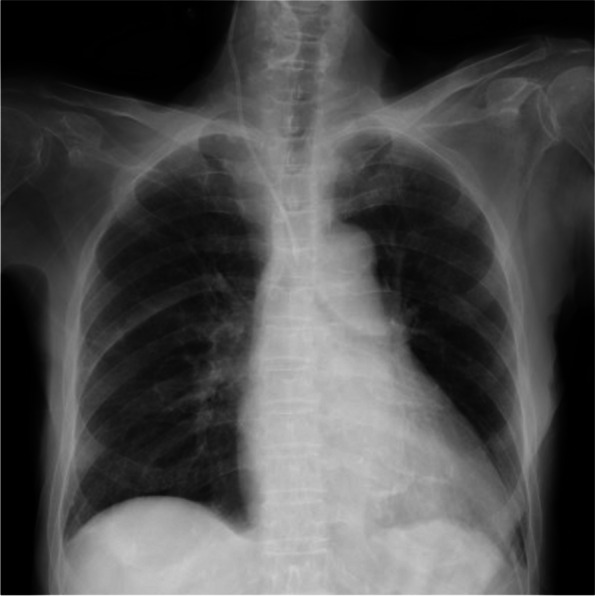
Postprocedural chest roentgenography. The catheter tip appears to overlap the shadow of the trachea where the right brachiocephalic vein or aortic arch exists
